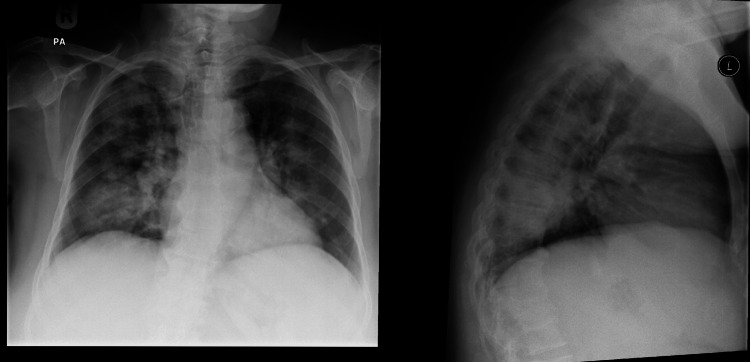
Chest x-ray showing bilateral air space disease with heterogenous airspace opcity seen in both lungs, more in the right side
